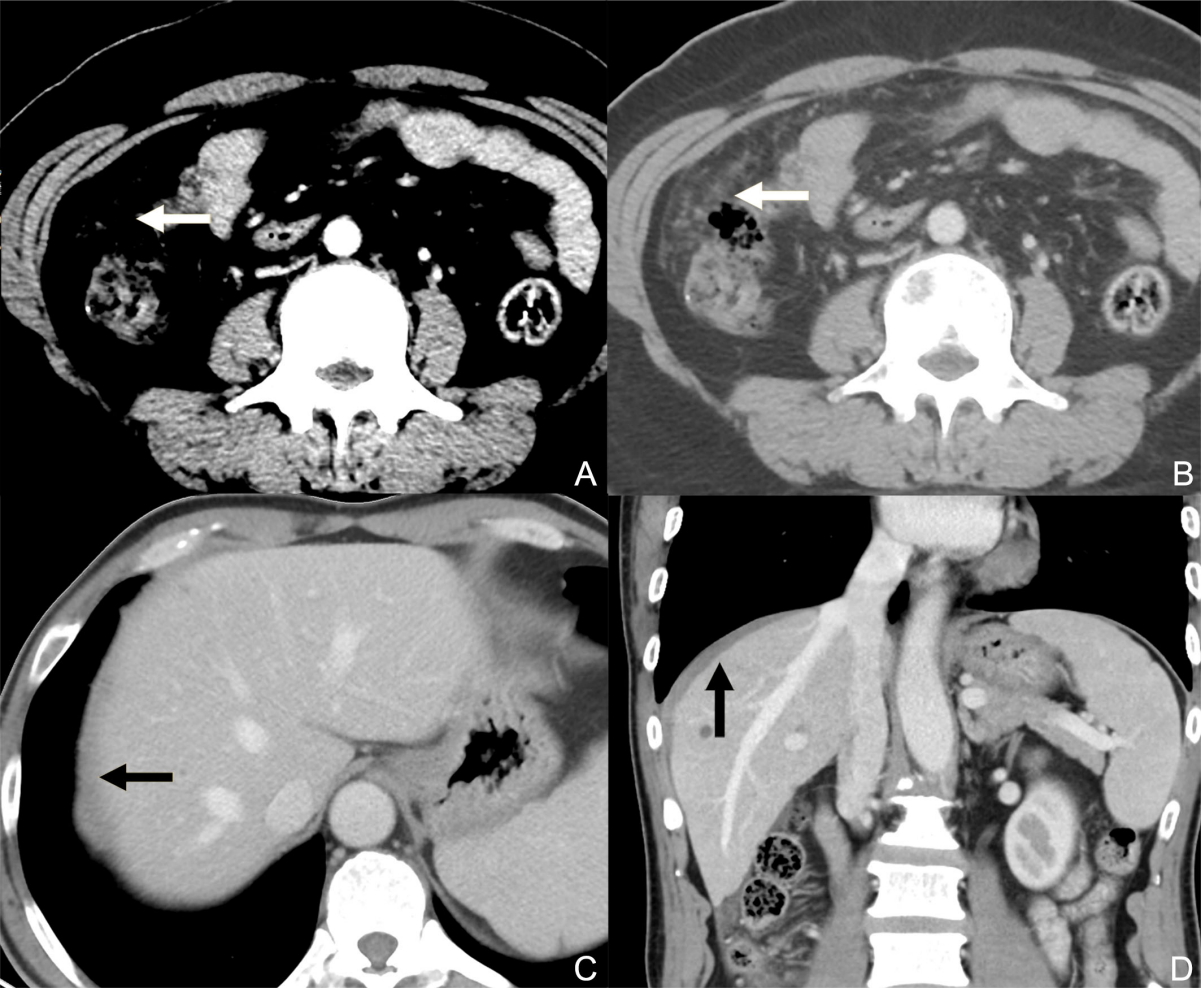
Contrast-enhanced CT axial plane with a narrowed window width (A) does not reveal peritoneal metastasis, while that with a wide window width (B) clearly reveals discrete nodules on the greater omentum. Contrast-enhanced CT axial plane (C) presents the equivocal thickening of the superior parietal peritoneum (black arrow) caused by the partial volume effect. In the sagittal plane (D), the involvement of the superior parietal peritoneum can be better observed as an enhanced diffuse thickening (black arrow, compared with the contralateral superior parietal peritoneum).
